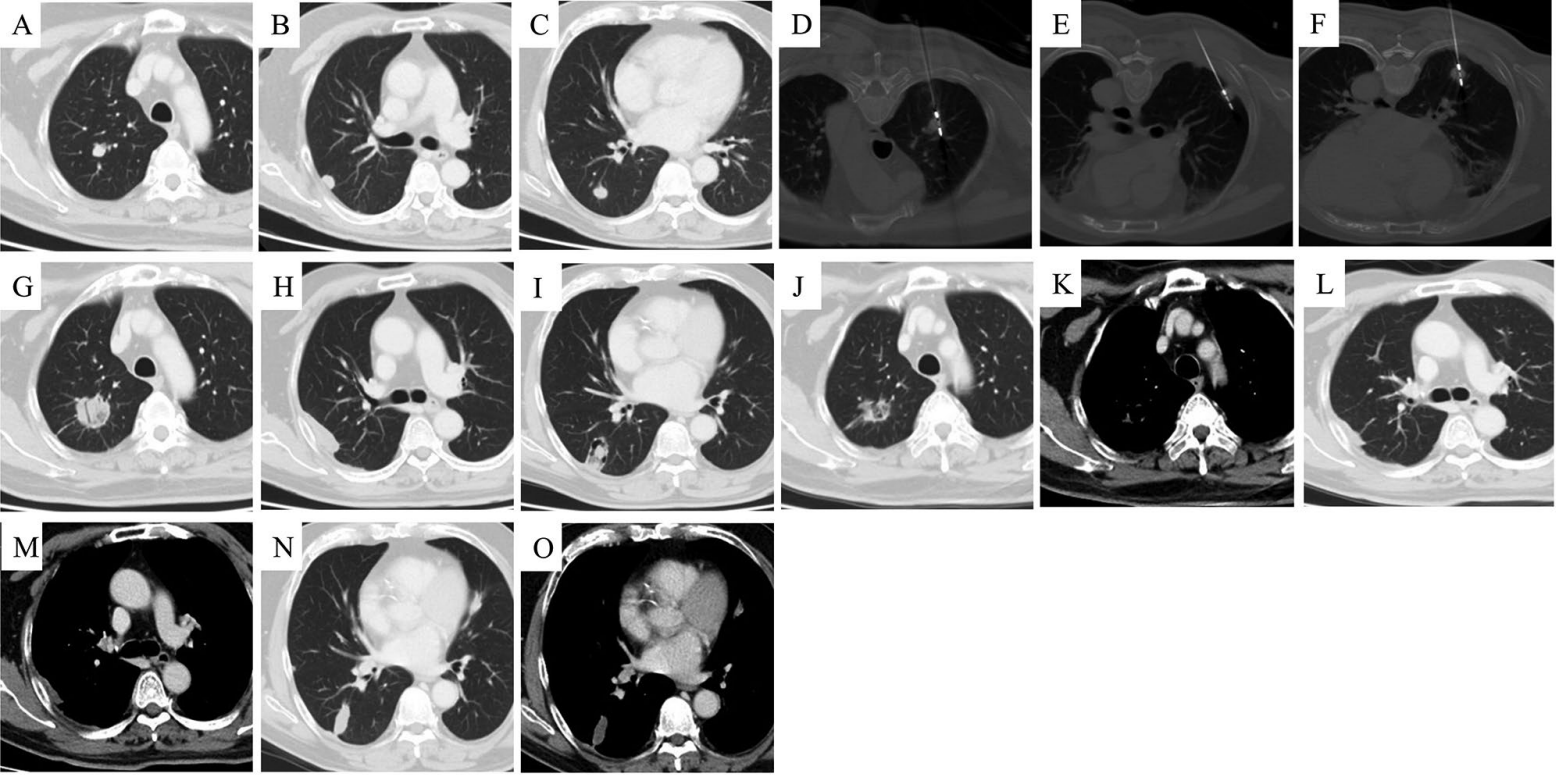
A 69-year-old male had three nodules in the right lung lobe for a month, detected nine months after primary hepatoma carcinoma surgery. A Nodule #1 (1.6 × 1.5 cm), and B nodule #2 (1.5 × 1.5 cm), were observed in the right upper lung lobe. C Nodule #3 (1.5 × 1.5 cm) was observed in the right lower lung lobe. D–F MWA was performed on nodules #1, #2, and #3 under CT guidance. G–I A month after MWA, exudative peripheral changes were observed surrounding all three lesions. J–O Nine months after MWA, the lesions shrank to fibrous cords with no enhancement. The efficacy was assessed to achieve complete ablation
